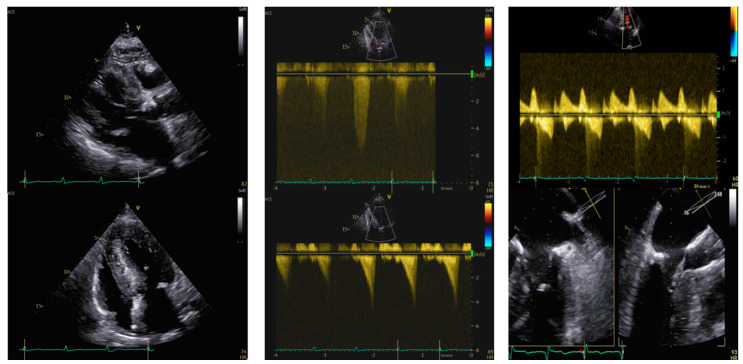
Transthoracic and transesophageal echocardiography in an 86-year-old woman before and after aortic valve stenosis percutaneous treatment and transcatheter alcohol septal ablation. Left panel: PLAX view (upper) and 4-chamber view (lower); IVS = 26 mm; LVEDVi = 45 mL/mq. Central panel: aortic valve peak velocity of 5.8 m/s (upper) with a mean gradient of 77 mmHg and dynamic LVOT gradient (lower) of 65 mmHg. Right panel—after TAVI and alcohol septal ablation: dynamic LVOT gradient (upper) of 20 mmHg but evidence of residual SAM of the anterior mitral leaflet (lower) by TEE. IVS: interventricular septum. LVEDVi: left ventricular end-diastolic volume indexed. LVOT: left ventricular outflow tract. SAM: systolic anterior motion. TAVI: transcatheter aortic valve implantation.
